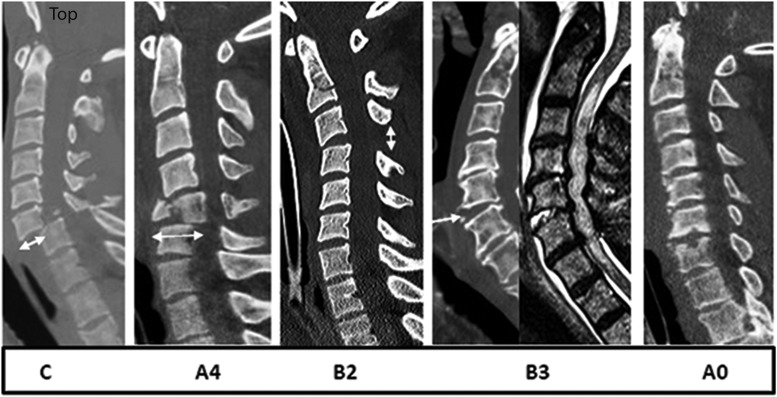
Midsagittal subaxial CT and MRI cuts indicating morphology according to AOSpine Subaxial Cervical Spine Classification System.^34^ C, translation rotation and highly unstable injuries; A4, compression burst fractures; B2, flexion distraction without translation; B3, extension distraction without translation; A0, no imaging evidence of fracture dislocation.
